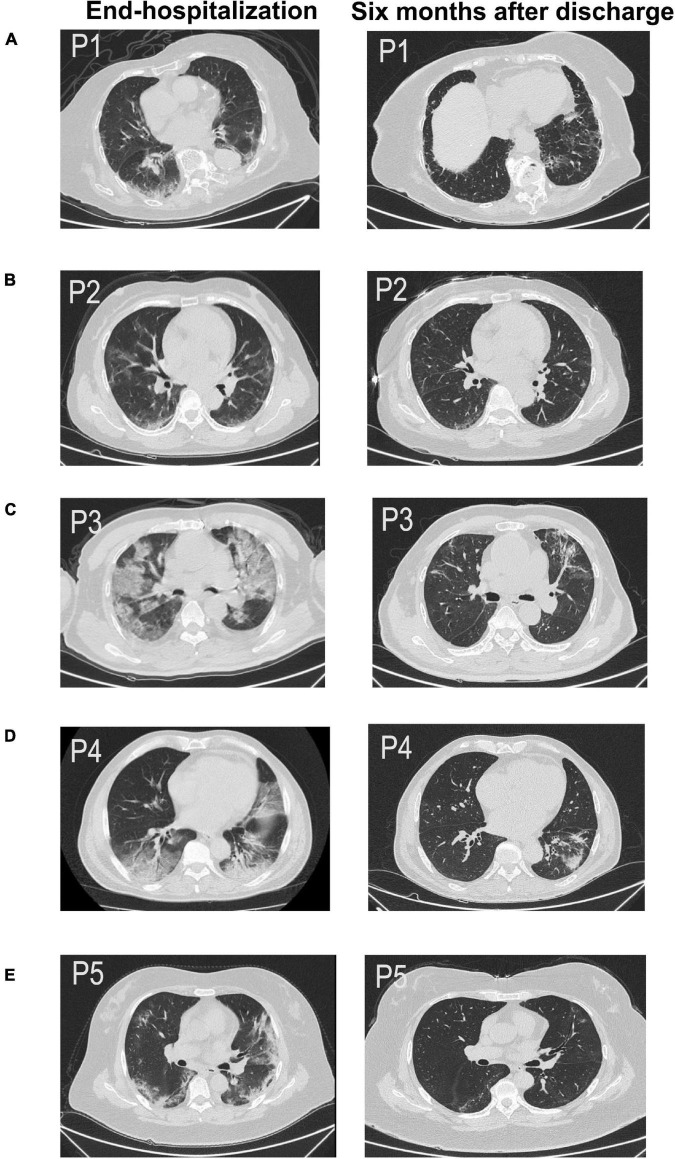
Chest CT scan of five patients (A–E) across two time periods, such as end-hospitalization and after 6 months discharge. All of them were pulmonary sequelae after 6 months of discharge (P1–P5). (A) CT image of a 51-year-old woman (P1) showing fiber streak shadow, reticulation, and tractive bronchiectasis 6 months after discharge. (B) CT image of a 57-year-old woman (P2) showing fiber streak shadow, reticulation, and tractive bronchiectasis 6 months after discharge. (C) CT image of a 76-year-old man (P3) showing ground glass opacity, fiber streak shadow, reticulation, and tractive bronchiectasis 6 months after discharge. (D) CT image of a 63-year-old man (P4) showing fiber streak shadow, reticulation, and bronchovascular bundle distortion. (E) CT image of a 65-year-old woman (P5) showing ground glass opacity, fiber streak shadow, and reticulation.
